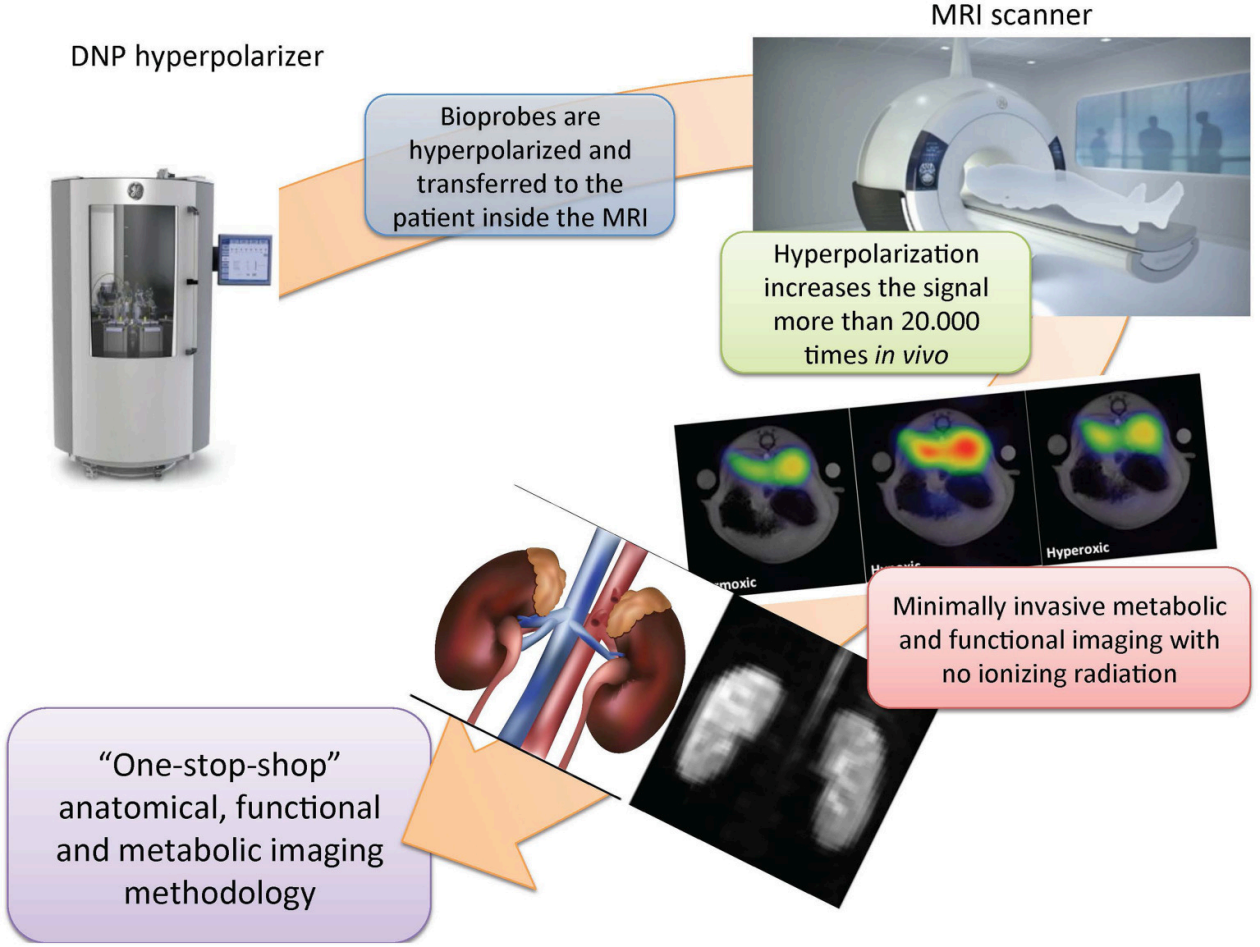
Dissolution dynamic nuclear polarization magnetic resonance imaging—a “one-stop-shop” methodology. The biomarker is hyperpolarized and transferred by intravenous injection to the patient inside the clinical scanner. This procedure increases the sensitivity of the measurements by more than 20,000 times, and enables the direct interrogation of renal uptake and metabolic turnover. Standard clinical magnetic resonance imaging (MRI) capabilities are simultaneously available and provide a very versatile and sensitive diagnostic modality that is free of ionizing radiation and thereby ensures patient safety. DNP, dissolution dynamic nuclear polarization.
